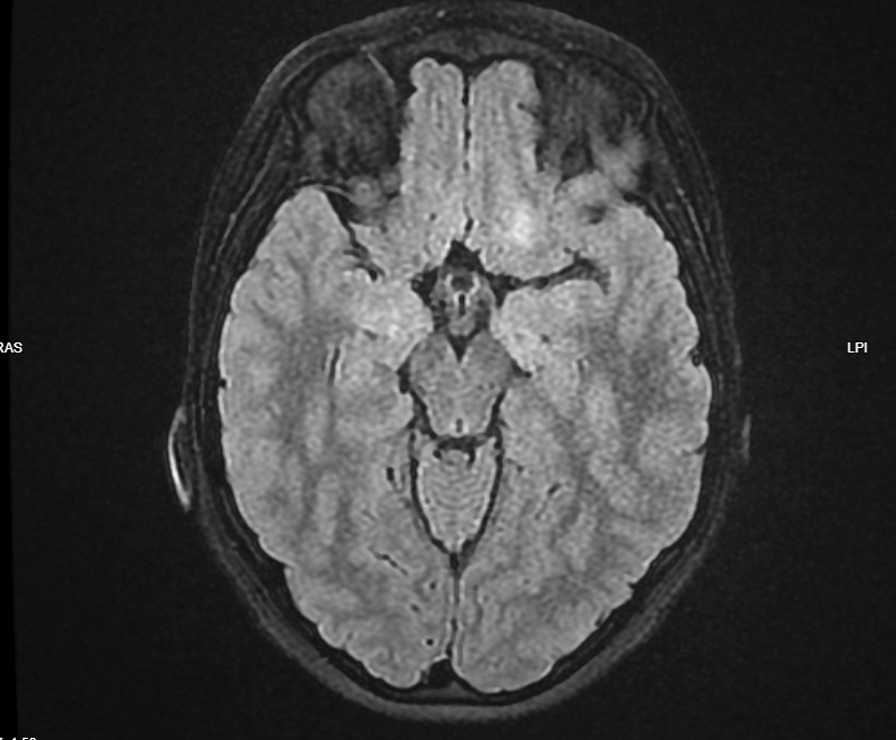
Image of a brain MRI scan, Ax T2 Flair. Image of a brain MRI scan performed during acute phase of a disease showing brain cortex thickening with hyperintense signal in both hippocampi and another 10 mm hyperintense focus at the base of the right frontal lobe
